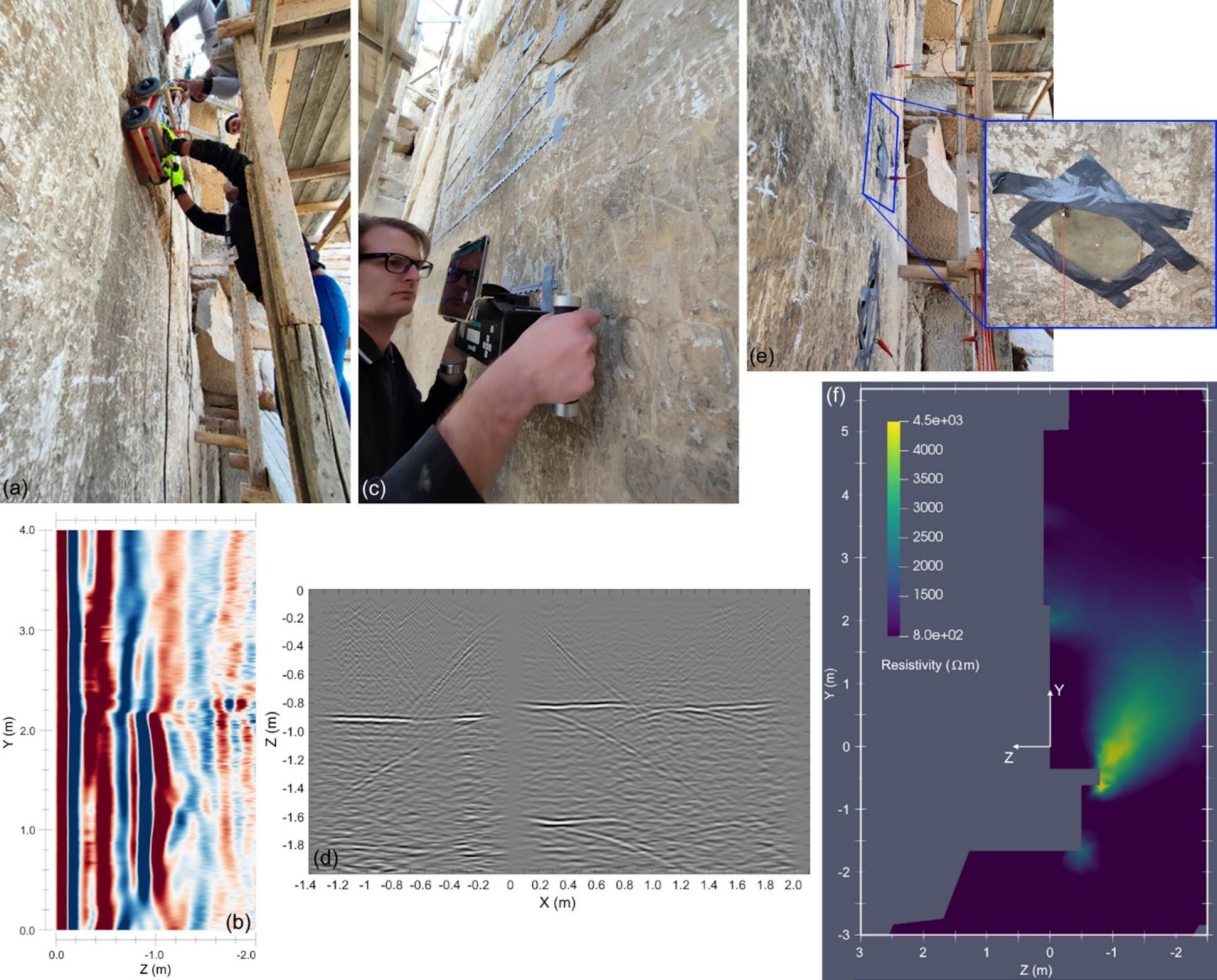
Photos and sample reconstructed images from the three NDT techniques used in this work: GPR—(a) Photo of ongoing vertical measurement with ground-coupled instrument and (b) sample reconstructed GPR image (200 MHz antenna) for vertical profile V1 located at X = 0.4 m; UST—(c) Photo of ongoing horizontal measurement with ultrasonic shear-wave pulse echo array instrument and (d) sample reconstructed UST image for horizontal profile H1 located at Y = 1.4 m; ERT—(e) Photo of electrodes arranged vertically on a straight line and (f) sample reconstructed ERT slice from a 3D model for vertical profile V1 located at X = 0.4 m. For profile locations see Fig. 1b. A collection of all reconstructed images can be found in the Appendix, Figures A1 through A3.
